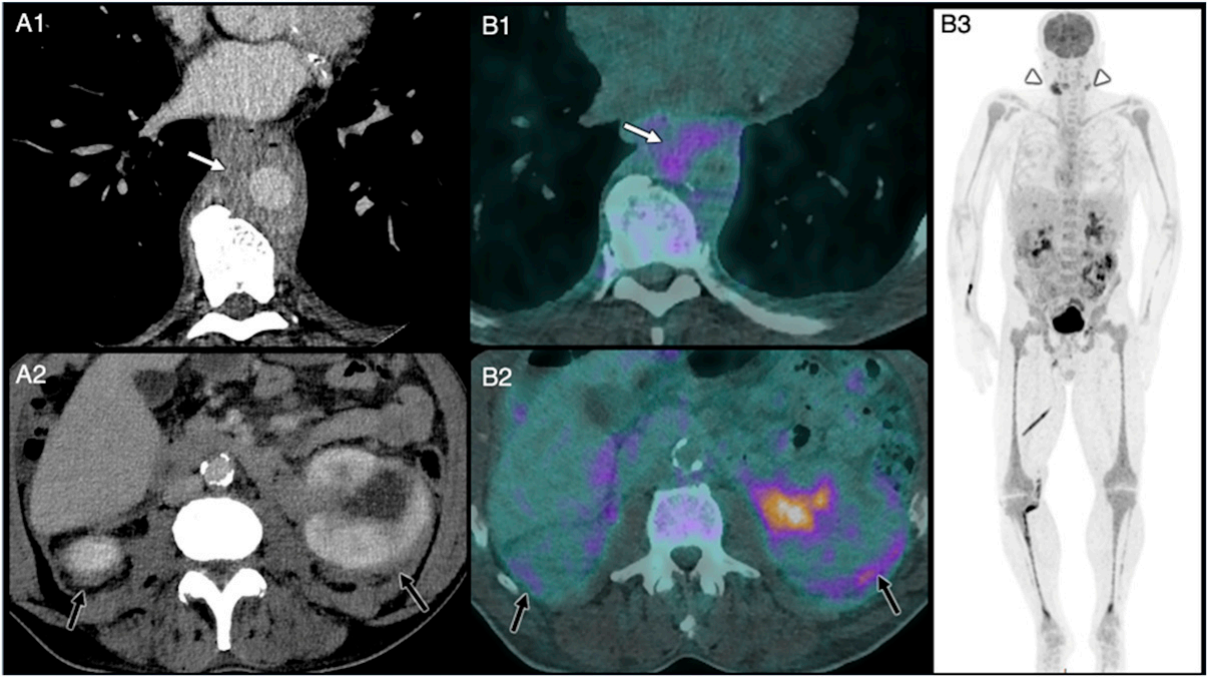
Axial FDG PET-CT with contrast-enhancement images acquired 10 days after the initial CT-scan showing periaortic wall thickening (arrow, A1), which is also hypermetabolic (arrow, B1). Peri-renal and peripyelic infiltration, giving a ‘hairy kidney’ appearance, is also seen (arrow, A2), with hypermetabolism of the area (arrow, B2). A maximum intensity projection of the PET-CT shows numerous hypermetabolic areas that appear dark, with notable neck lymphadenopathy (arrows, B3).
